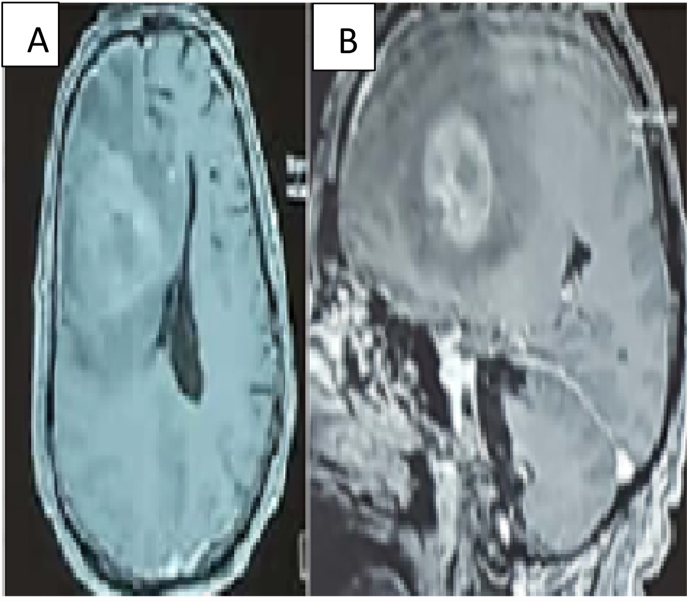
MRI: A: axial image, B: sagittal image right fronto-parietal formation surrounded by a peri-lesional edema. .
